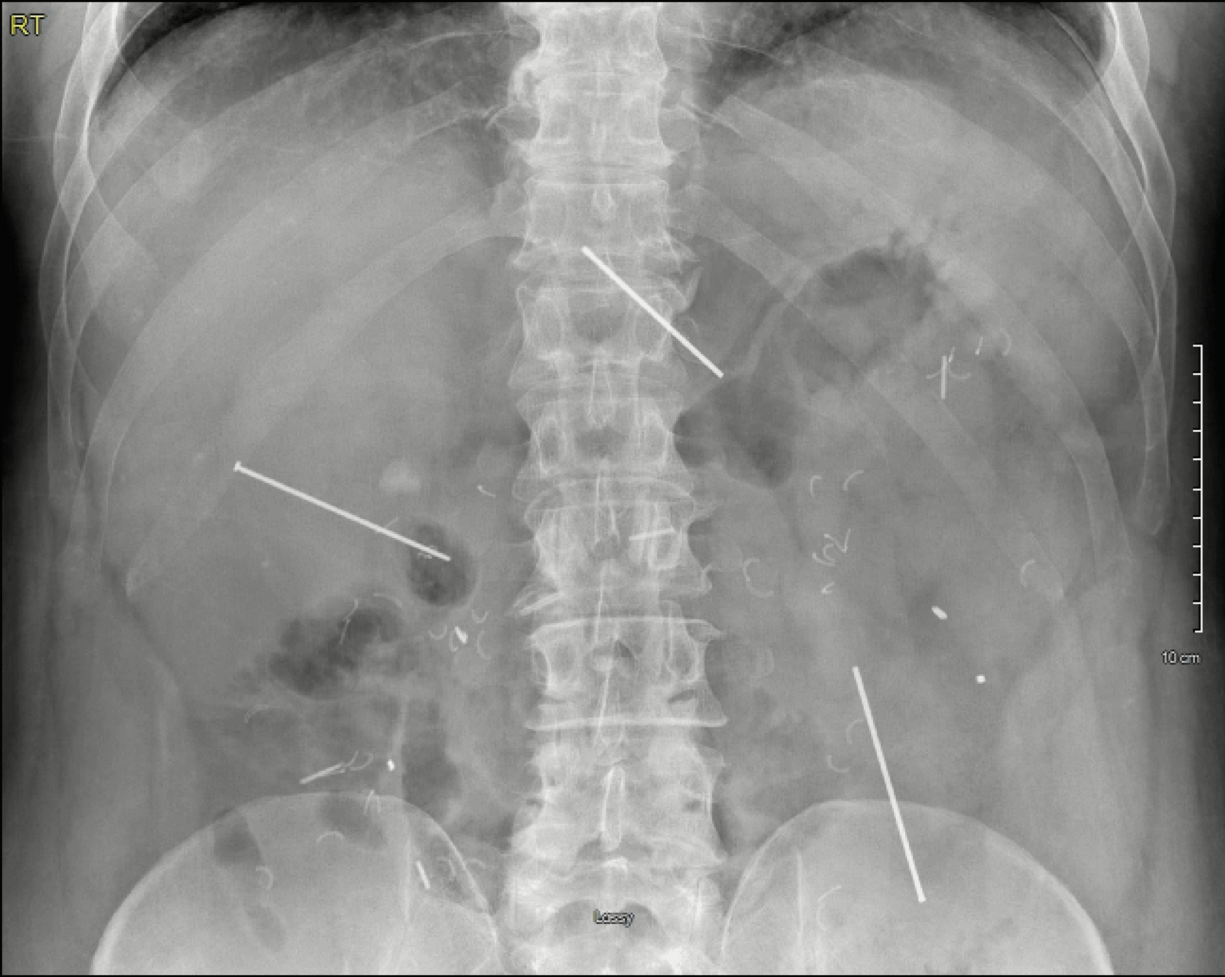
Three nails within the abdominal cavity on abdominal X-ray
